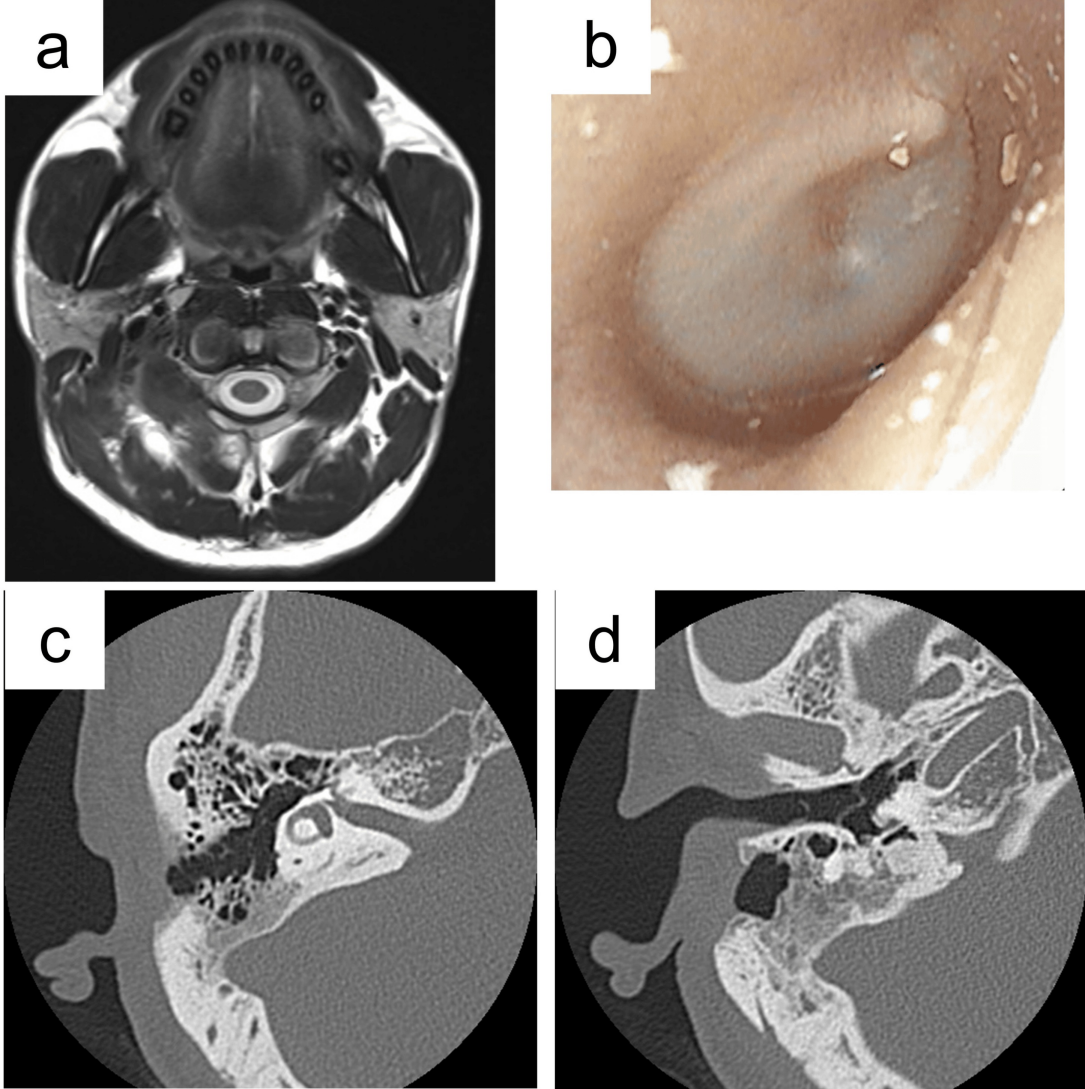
Follow-up at 1.5 and 6 months after surgery. (a) Axial T2-weighted MRI obtained 1.5 months postoperatively, demonstrating resolution of cervical muscle inflammation and collapse of the abscess cavity.
